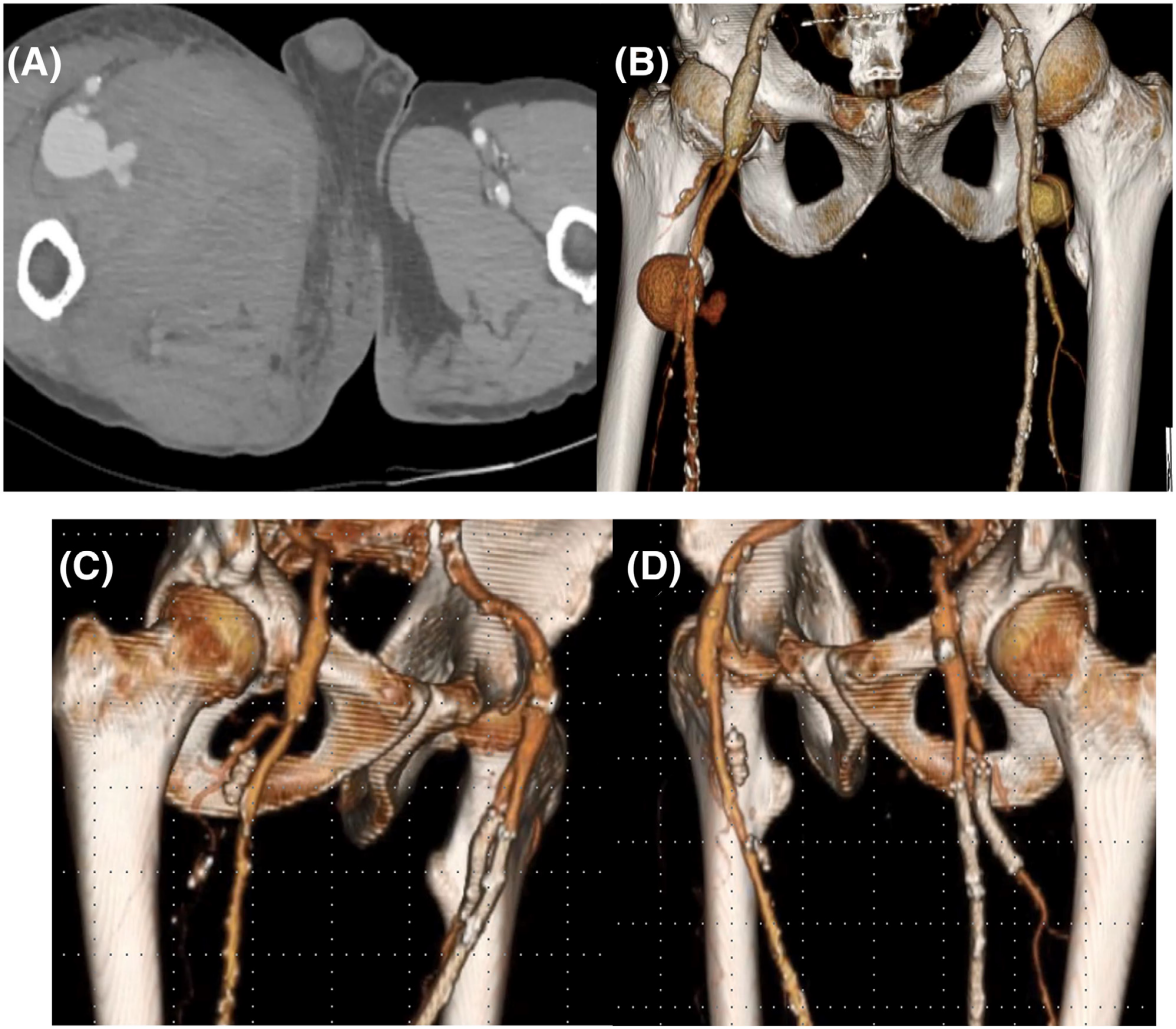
CT revealed bilateral DFAA and ruptured right‐sided aneurysm (A, B). Postoperative CT showed exclusion of DFAA blood flow and patent stent grafts (C, D).
